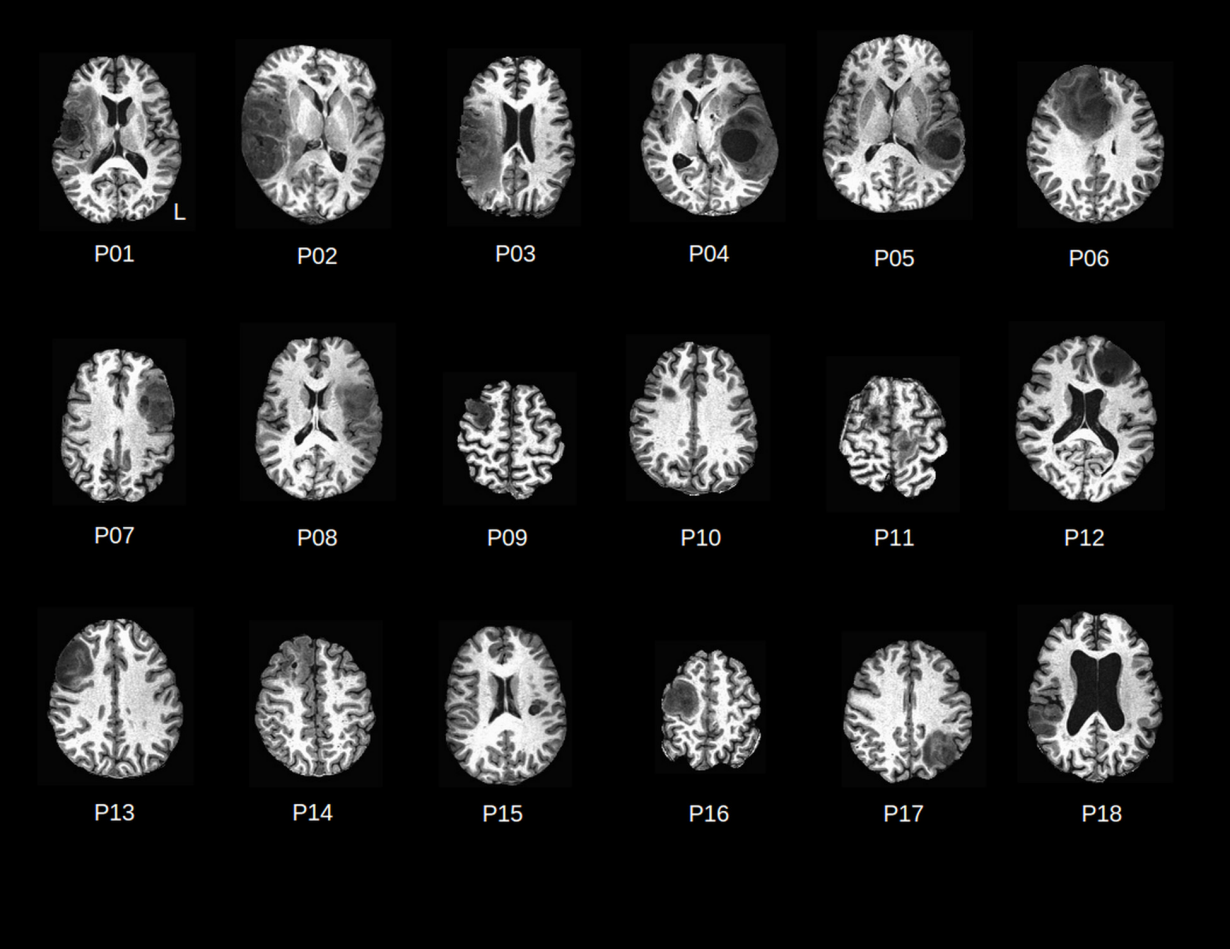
T1-weighted axial MRI slices displaying patient brain tumor anatomy (P01-P18).
